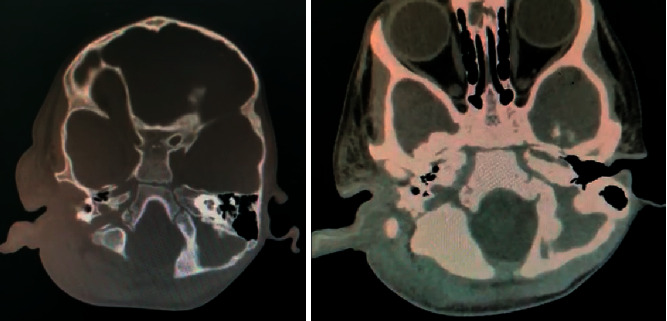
CT scan showing diffuse left side periauricular swelling.
